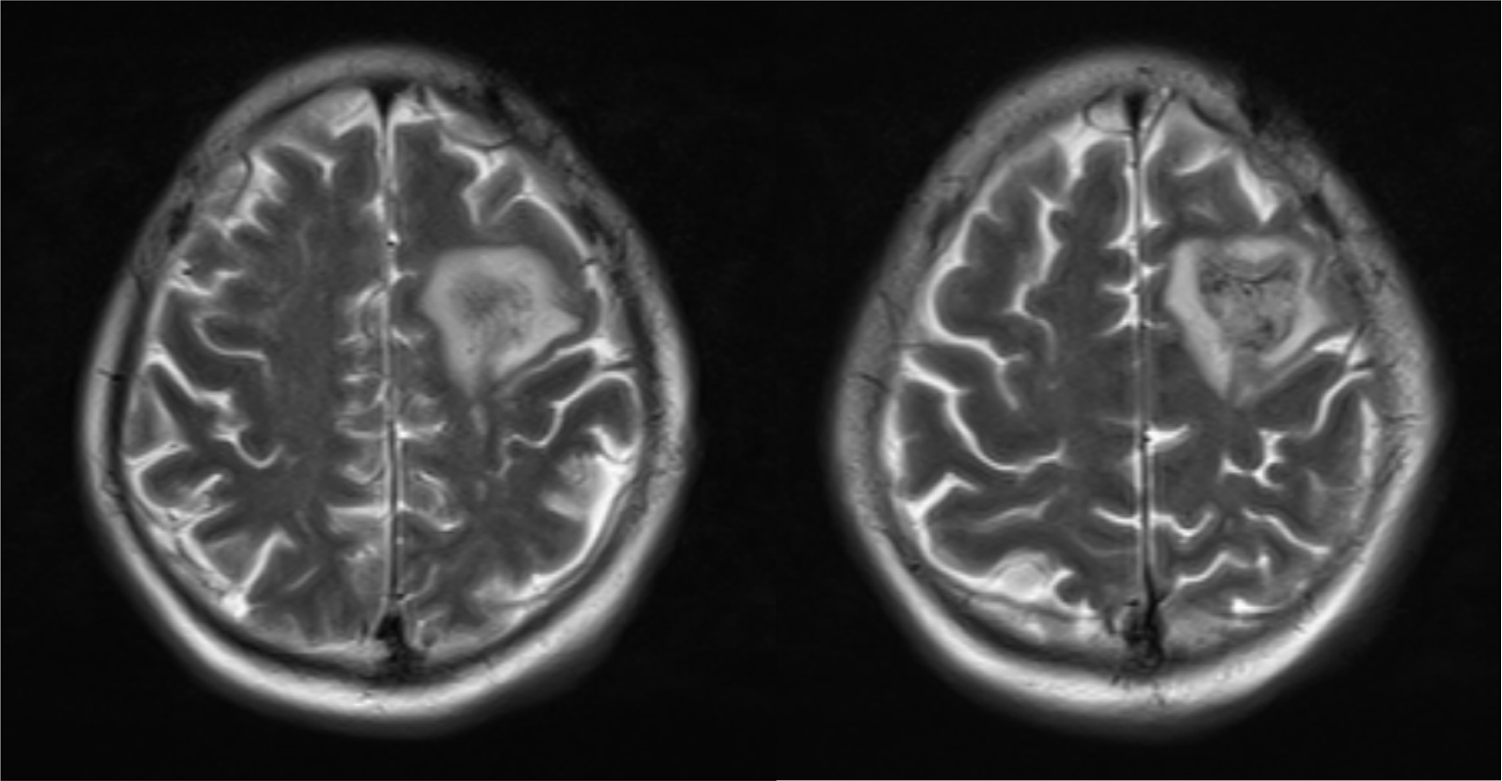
Head MRI shows abnormal signals in the left frontal lobe. Combined with the medical history, hemorrhagic infarction caused by bacterial embolism was considered. No obvious abscess cavity is seen, and the hemorrhage is in the subacute stage.
